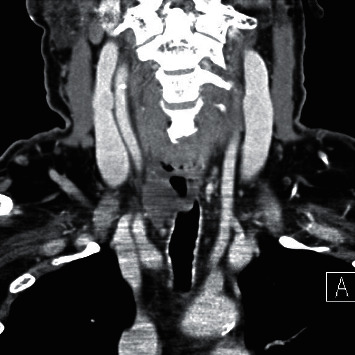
CT scan of the neck after 25 doses of pembrolizumab (coronal image).
